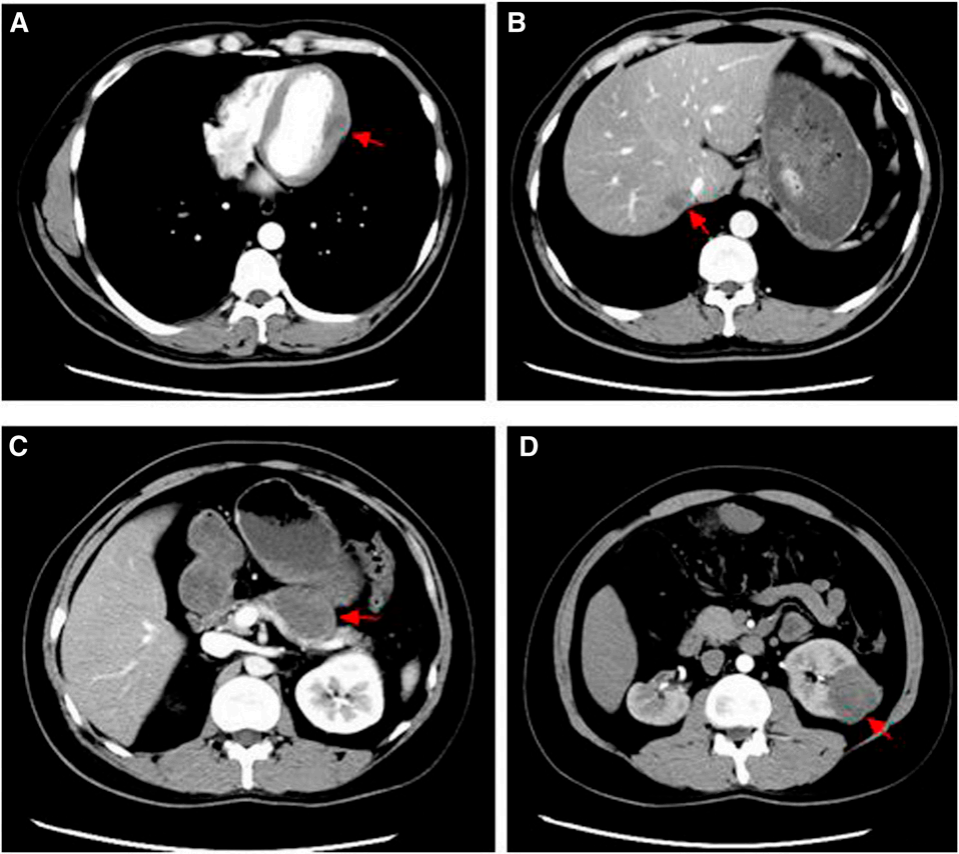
(A–D) Posttreatment computed tomography (CT) images of the left ventricle (CT value: 26 to 40 HU) (A), liver (CT value: 27 to 49 HU) (B), pancreatic tail (CT value: 26 to 51 HU) (C), and left kidney (CT value: 19 to 49 HU) (D). This figure appears in color at www.ajtmh.org.
